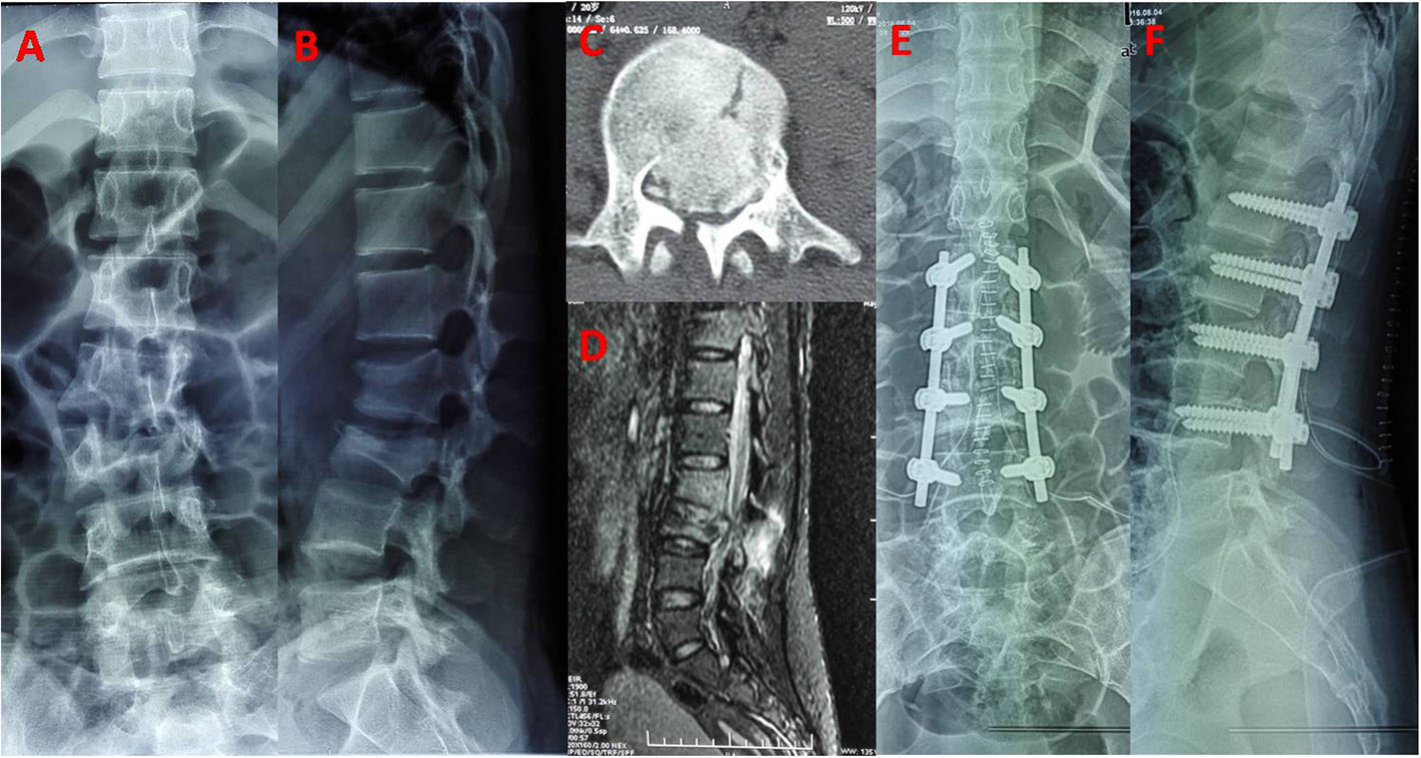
a, b. A 20-year-old woman with an L3 burst fracture and incomplete paralysis. c. Axial CT image shows comminution and canal encroachment. d. Sagittal MRI shows PLC injury. e, f. Posterior monoaxial pedicle screw fixation was done from L1 to L4. The kyphotic deformity was corrected and fractured vertebra height was restored
